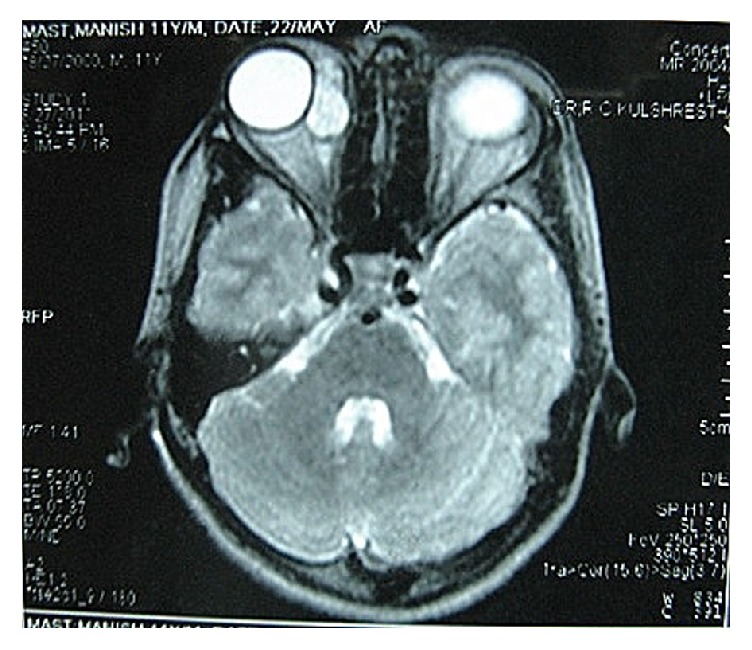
MRI scan of orbit MRI of the orbit revealed a well-defined mass of approximately 23 × 13 mm along the medial rectus muscle.
